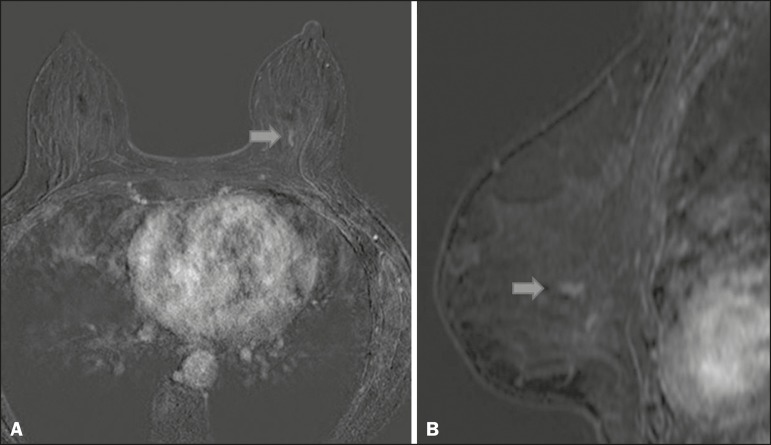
Contrast-enhanced, high-resolution MRI. Axial sequence, with digital
